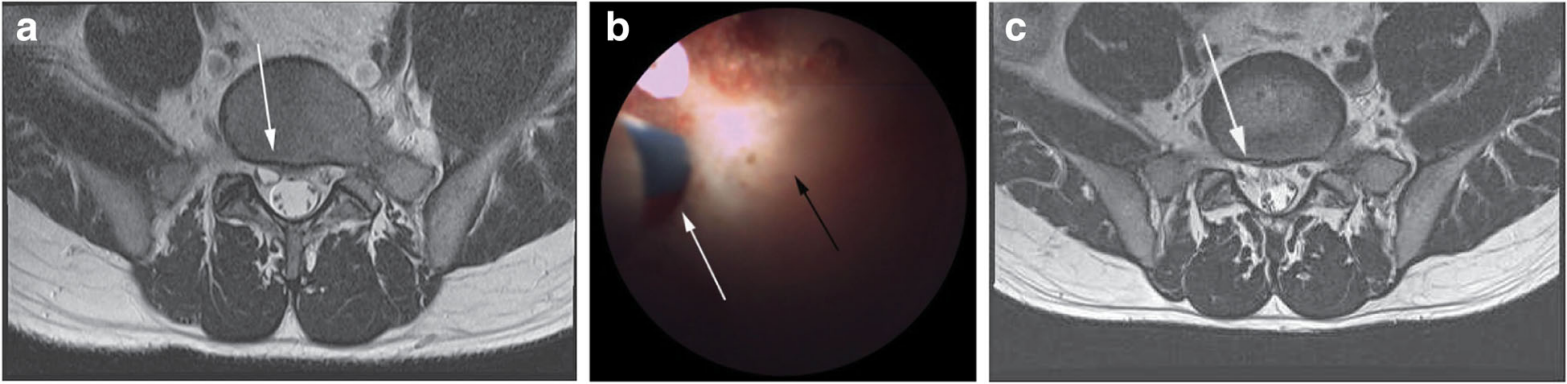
a T2-weighted axial magnetic resonance (MR) image shows a cystic mass (arrow) originating from the disc and compressing the traversing right nerve root. b Epiduroscopic image shows the laser electrode inserted (white arrow) into the cyst (black arrow). c Post-operative T2-weighted axial MR image shows the decompressed cyst (arrow) and visualization of the L5 traversing root
